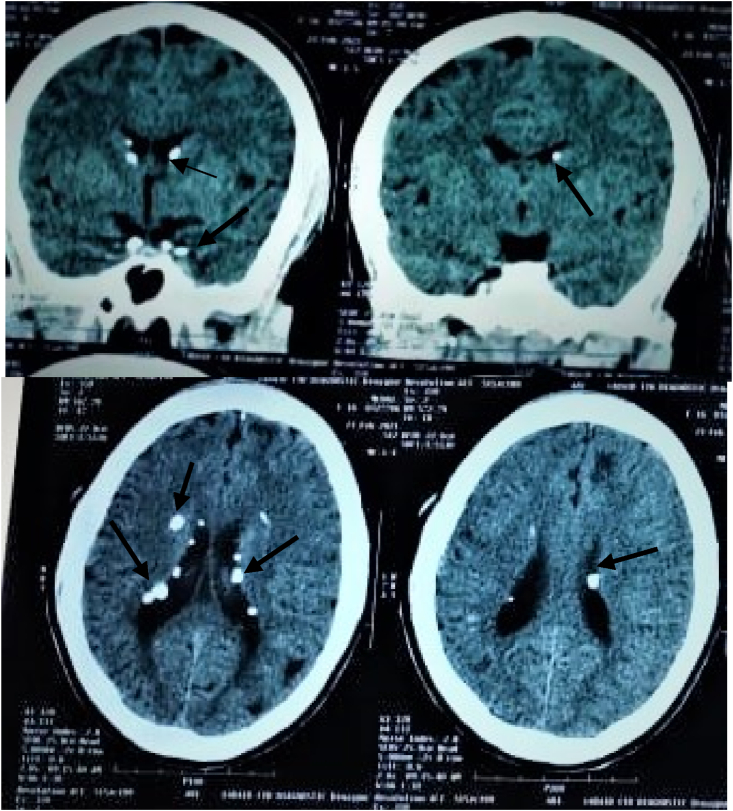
CT scan of head showing sub ependymal nodules.
